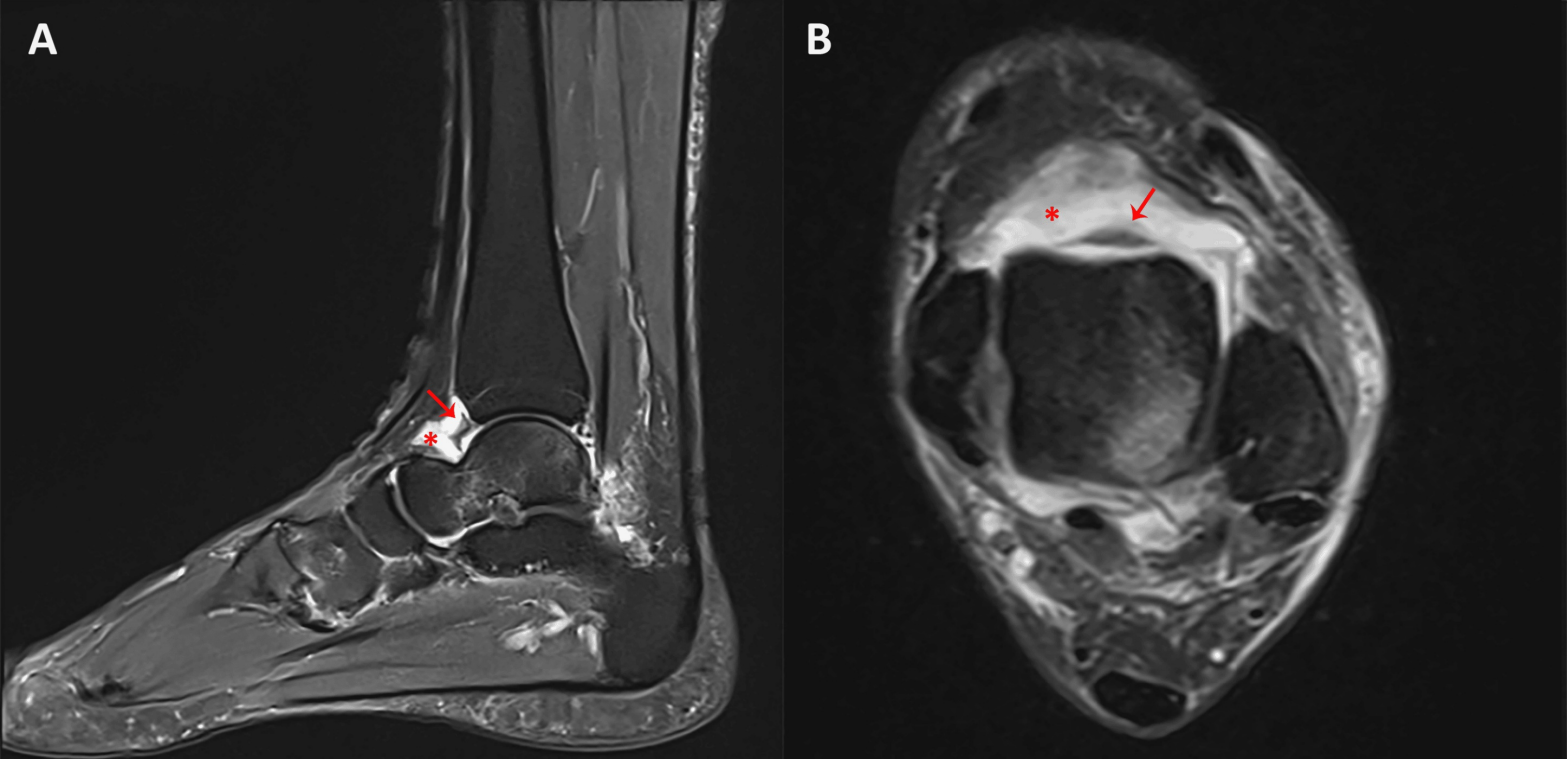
Sagittal and axial proton density fat-saturated MRI demonstrating intra-articular effusion and fibrous band formation. (A) Sagittal view showing a linear fibrous band traversing the anterior talocrural recess (red arrow) and associated joint effusion (red asterisk). (B) Axial view confirming the presence of the fibrous band (red arrow) and intra-articular fluid accumulation (red asterisk).
